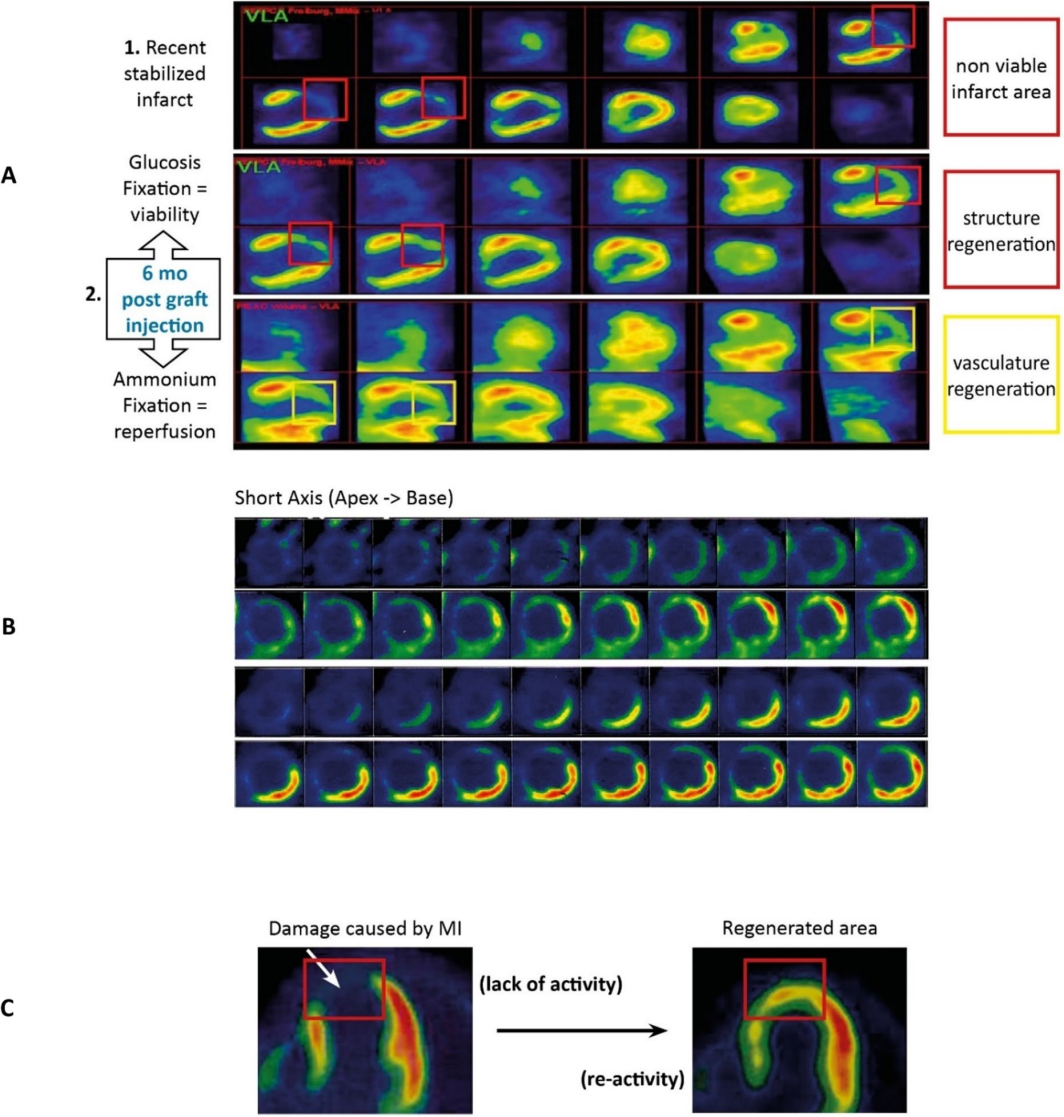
PetScan examinations of Patients 2, 4 and 5. A: Petscan of Patient 2. Upper raw: large non viability area (red square) in the left ventricle anterior wall before cells injection. Middle raw: cardiac tissue regeneration 6 months after cells injection, objectified by fixation of ^18^Fi-FDG into what was the dead area (red square). Lower raw: fixation of ^201^Ti-chloride 6 months after cells injection, showing the revascularization of the initially dead area (yellow square). B: Patient 4 ^18^Fi-FDG PetScan. Upper raw, before cells injection: non-viability of the myocardium involving the apex, almost all the anterior wall, the septum and the posterior wall from the apex to the middle of the ventricle. Lower raw, 1 year after cells injection: improvement of the viability in the basal parts of the anterior wall and of the septum, and in all dorsal wall. C: ^18^Fi-FDG PetScan images of Patient 5. Left: After AMI and before cells injection, the infarcted zone is inactive. Right: the same zone is again viable 1 year after cells injection
